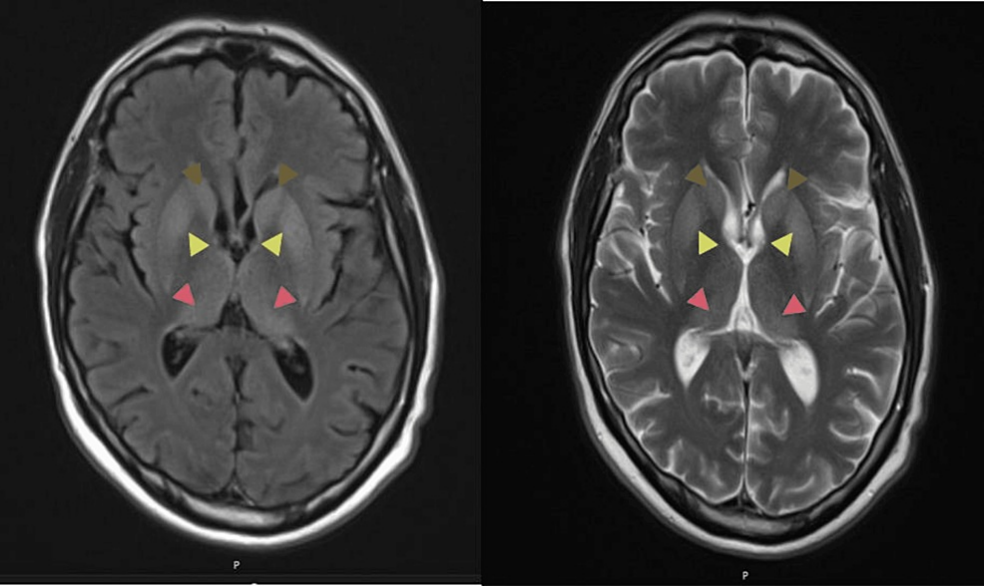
MRI images of the patient Pathological T2/FLAIR high signal on MRI within the deep grey matter, in particular the thalamus. Hyperdensities can be seen in the caudate nucleus bilaterally (marked by brown arrows), in the putamen nucleus of the thalamus bilaterally (marked by yellow arrows), and bilaterally in the pulvinar thalamic nuclei (marked by red arrows). The bilateral hyperdensities of the pulvinar nuclei are classically known as the 'pulvinar sign'.
